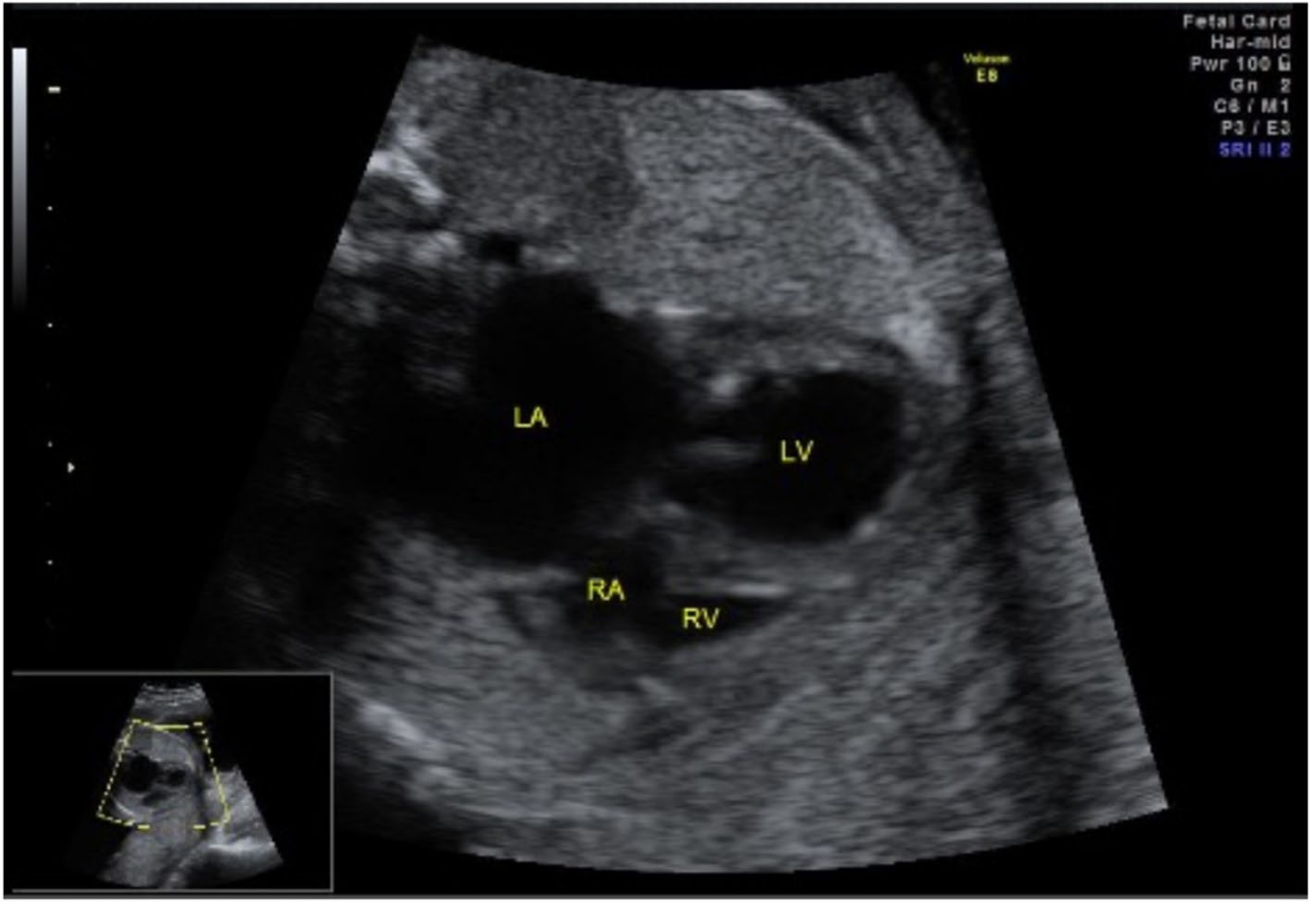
Fetal echocardiogram images at 25 weeks of gestation. Fetal echocardiogram in a four-chamber view demonstrating left atrial (LA) enlargement with bowing of the atrial septum into the right atrium (RA). The right ventricle (RV) is compressed by the dilated left ventricle (LV)
